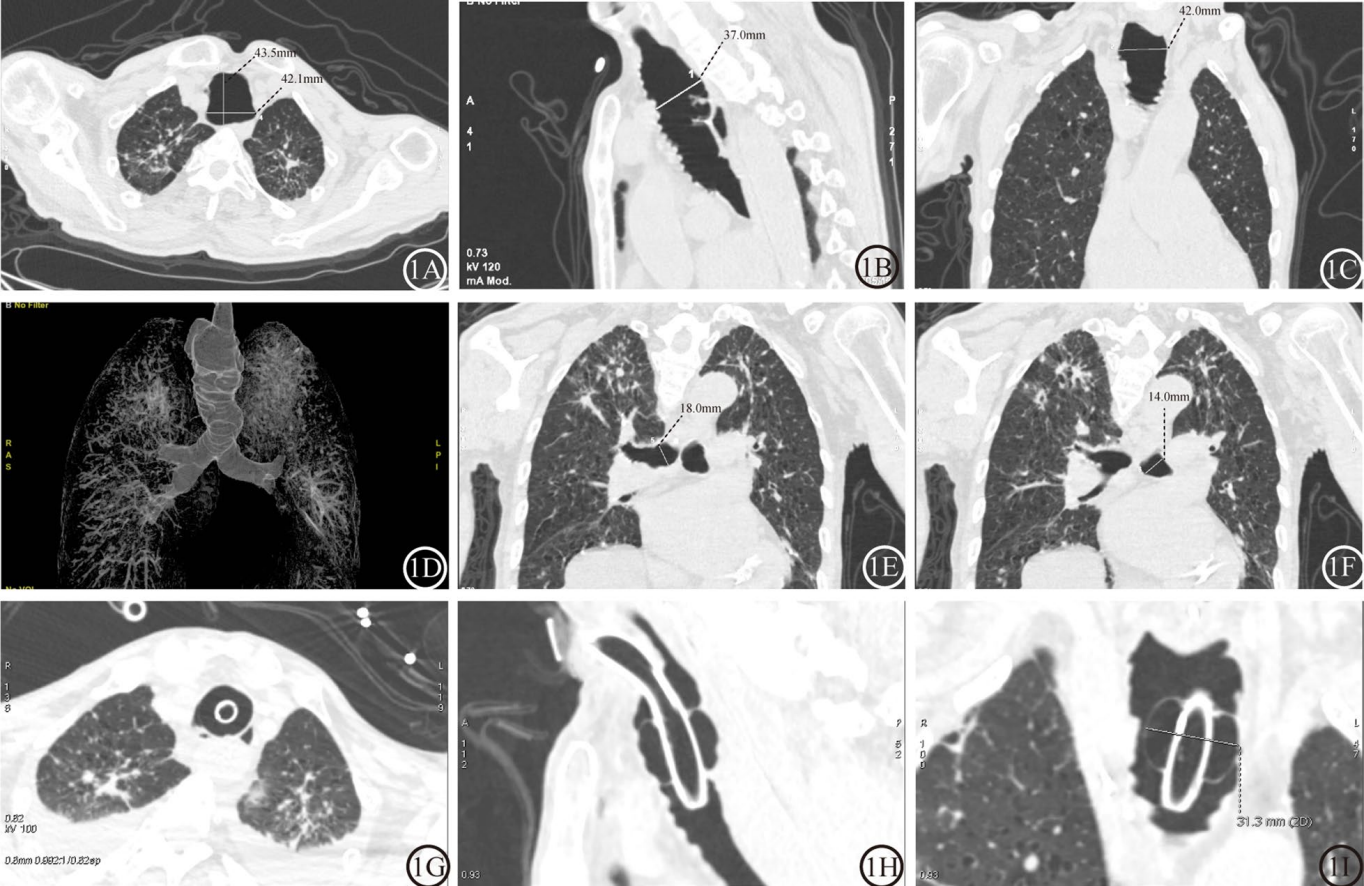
Multimodal CT evaluation of MKS (Case 1). A–C Axial, sagittal, and coronal views demonstrating pantracheobronchial dilation. D 3D airway reconstruction reveals main bronchial dilatation with pathognomonic features: multiple diverticula and cystic bronchiectasis. E, F Coronal oblique MIP images confirming bilateral mainstem bronchus dilation. G–I Posttracheostomy CT images revealing an inadequate cuff seal with air leakage, measured at the residual tracheal lumen.Abbreviations: MKS: Mounier–Kuhn syndrome; MIP, Maximum Intensity Projection; CT, computed tomography
